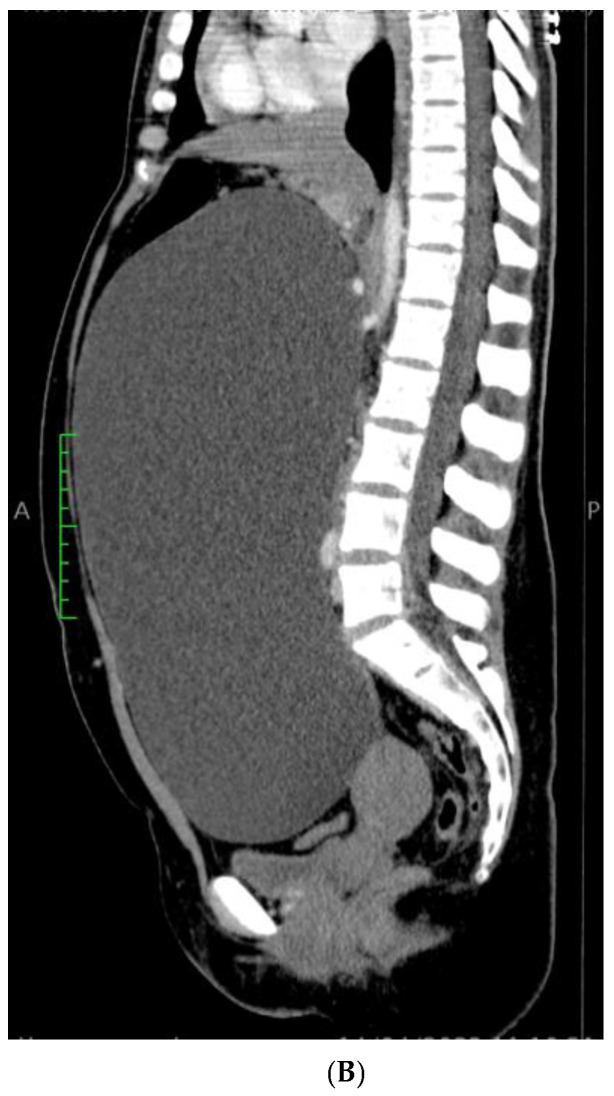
CT Scan: Gigantic ovarian cyst of 360/300/150 mm. (A) Sagittal section and (B) coronal section.
